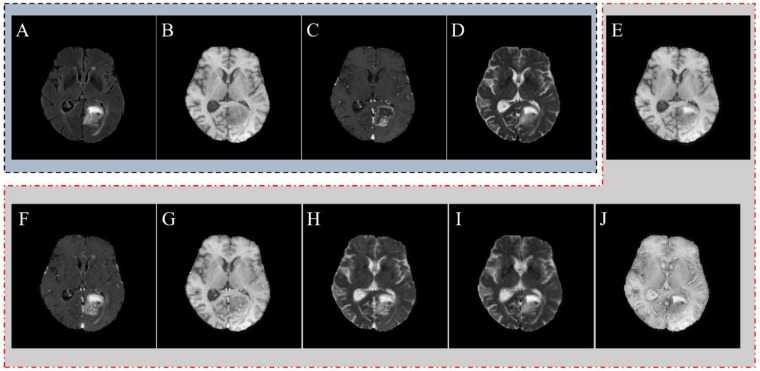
Original MRI and fused images of a high-grade glioma (HGG) subject in the BraTS dataset. (A–D) original MRI image weights, (E–J) fused images with the LRD medical image fusion algorithm. (A)—FLAIR. (B)—T_1_, (C)—T_1_Gd. (D)—T_2_, (E)—T_1_+FLAIR. (F)—T_1_Gd+FLAIR. (G)—T_1_Gd+T_1_. (H)—T_1_Gd+T_2_. (I)—T_2_+FLAIR. (J)—T_2_+T_1_.
